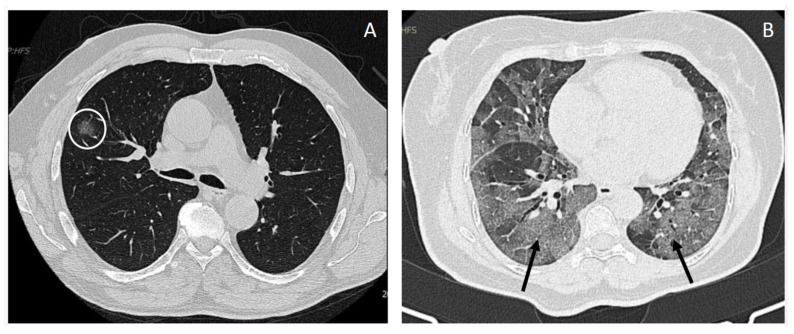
Representative axial chest CT images in lung window showing different degrees of pulmonary involvement. (A) Axial CT scan shows a ground glass opacity (white circle) with otherwise preserved lung parenchyma, representative of a mild form of COVID-19 pneumonia (CTSS = 2). (B) Axial CT scan shows extensive bilateral crazy paving pattern (black arrows) diffusely involving the lung bases, the middle lobe, and the lingula, suggestive of a severe form of COVID-19 pneumonia (CTSS = 22).
